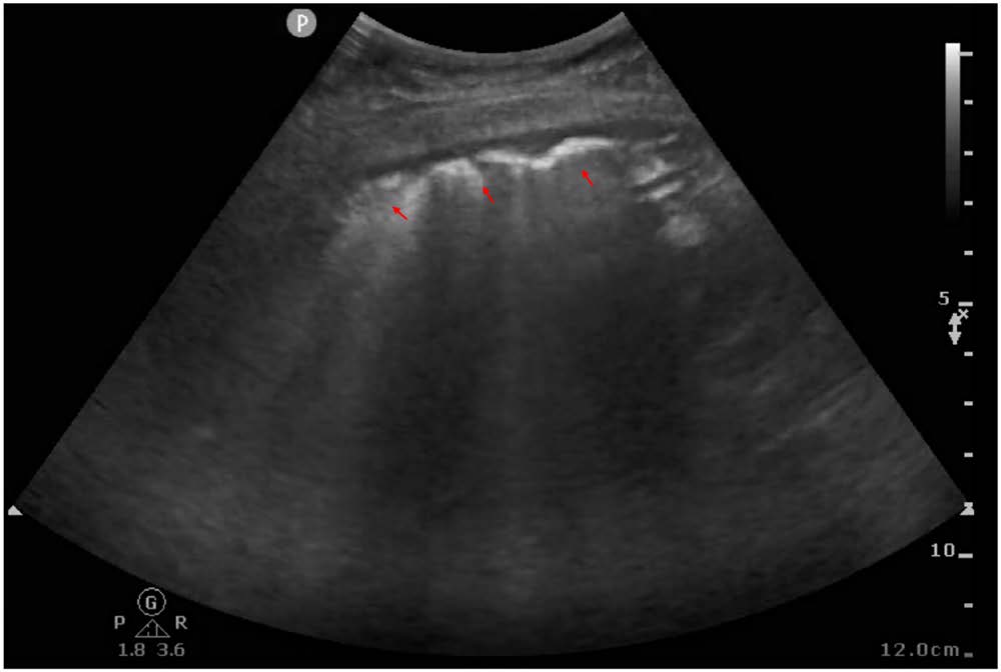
Bedside ultrasound image of chest examination revealed focal diffuse B-line chest wall with pleural slip (red arrows) on the left side.
